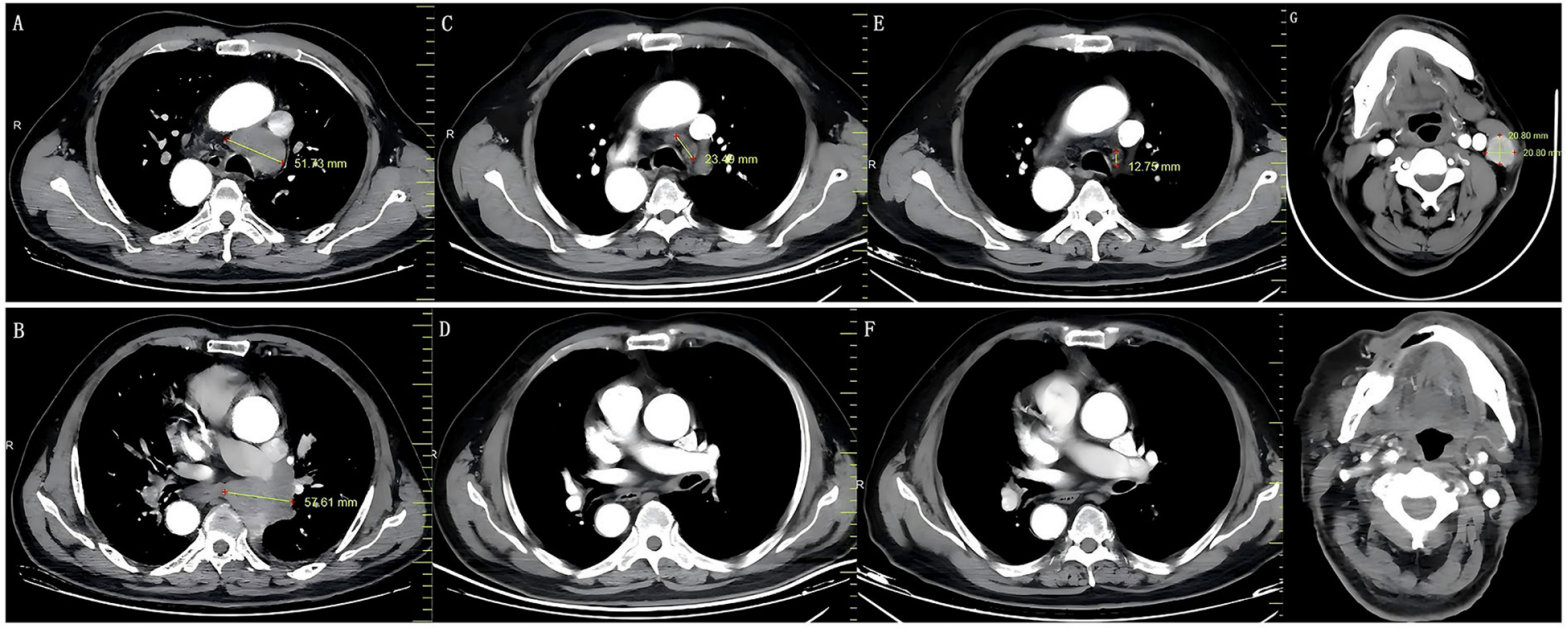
Therapeutic response assessment on chest enhanced CT. (a, b) Baseline imaging (July 1, 2025) of mediastinal lymph nodes and left hilar masses; (c, d) PR observed in the mediastinal lymph nodes and left hilar masses after chemoimmunotherapy (August 27, 2025); (e, f) CR achieved in the same regions after continued treatment (November 24, 2025); (g, h) The preoperative and postoperative imaging features of the left cervical LGMS.
